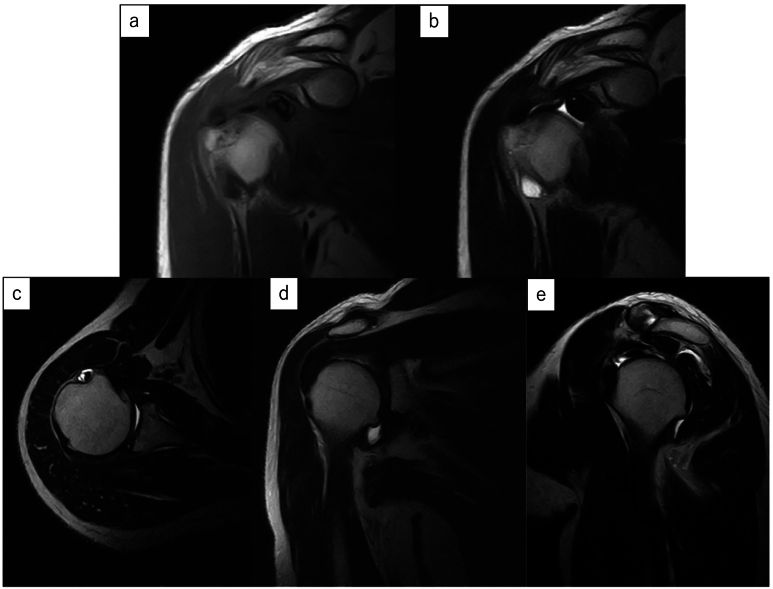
a, b MRI showed a loose body with isointensity in the center and low intensity at the periphery on T1-weighted images, and low intensity on T2-weighted images. a: T1WI: T1-weighted images. b: T2WI; T2-weighted images. c, d, e MRI did not reveal any obvious rotator cuff tears. c: Axial view d: Oblique Coronal view e: Oblique Sagittal view.
